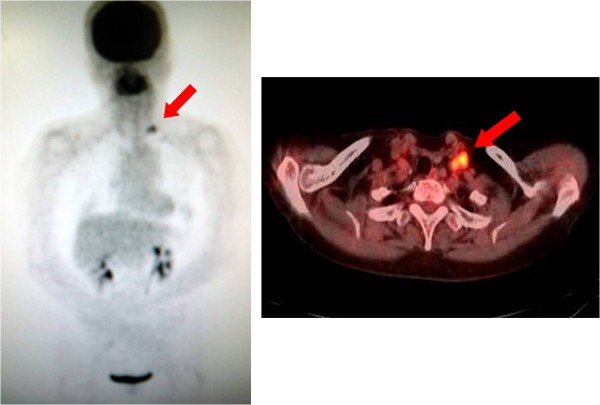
The positron emission tomography-computed tomography (PET-CT). PET-CT showed a single left supraclavicular lymph node (the arrow) with high uptake (SUV max 6.0). There were no other metastatic lesions in the body
